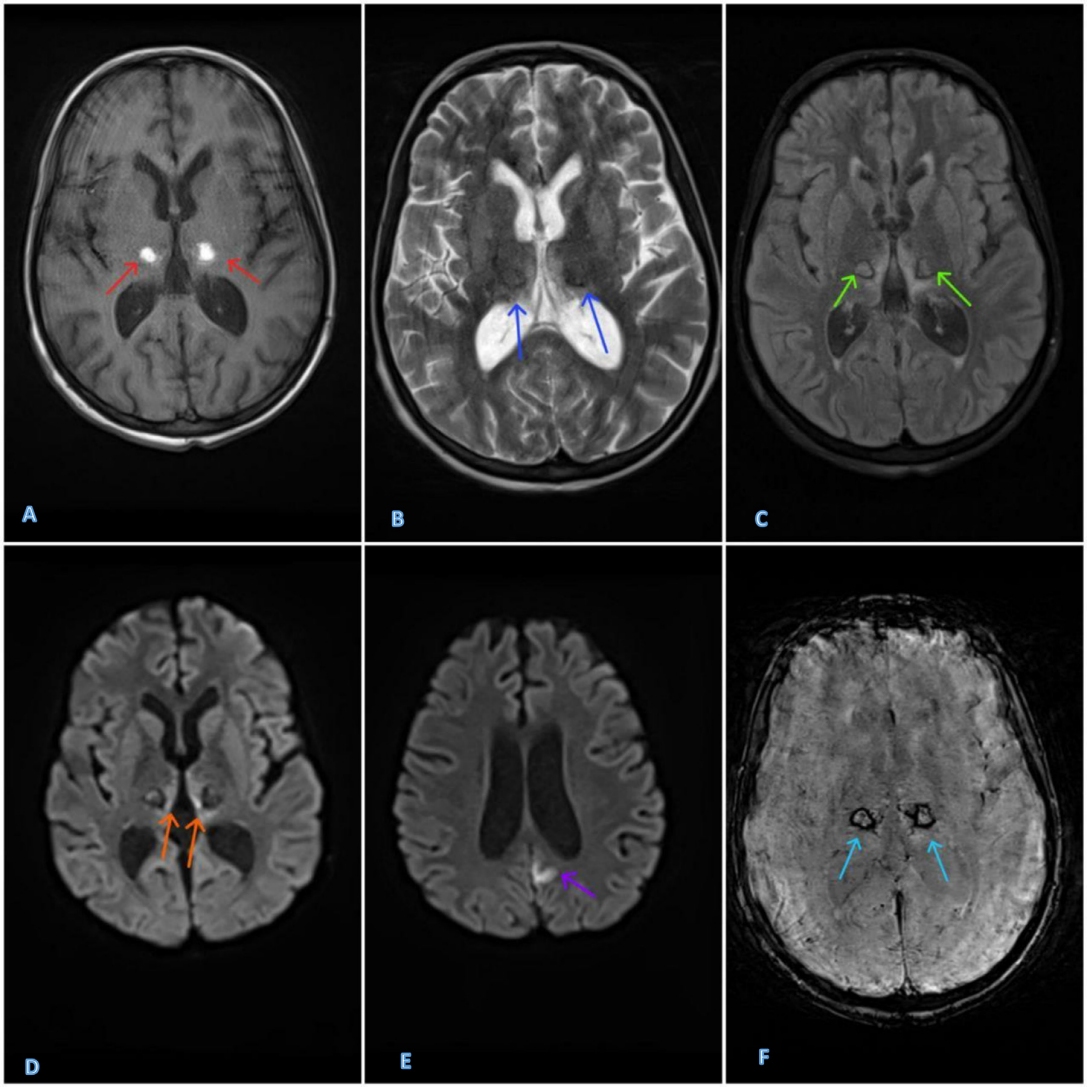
Brain MRI of a 14-year-old female patient showcasing the double doughnut sign, with evolution. There is a well-defined lesion in each thalamus, having T1 hyperintensity (A, red arrows), T2 hypo to isointensity (B, dark blue arrows), with fluid attenuated inversion recovery (FLAIR) and susceptibility weighted imaging (SWI) isointensity with a rim of hypointensity (C, green arrows and F, light blue arrows respectively). The paramedian parts of both thalami have restricted diffusion (D, orange arrows). There is also a small focus of cortical swelling and diffusion restriction in the parasagittal part of the left parietal lobe (E, purple arrow).
